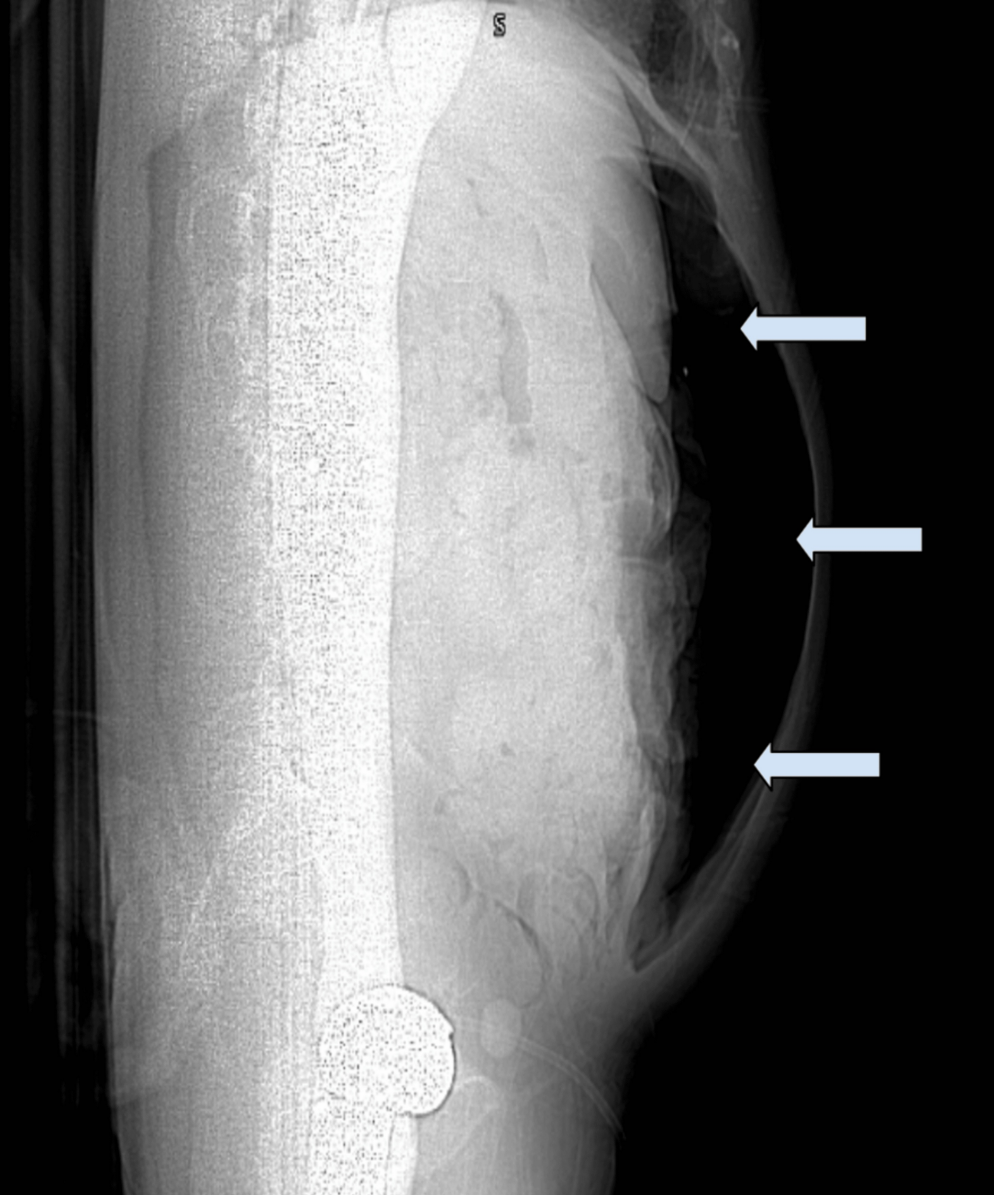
Sagittal abdominal X-ray of the patient showing signs of pneumoperitoneum, which prompted the emergent exploratory laparotomy
